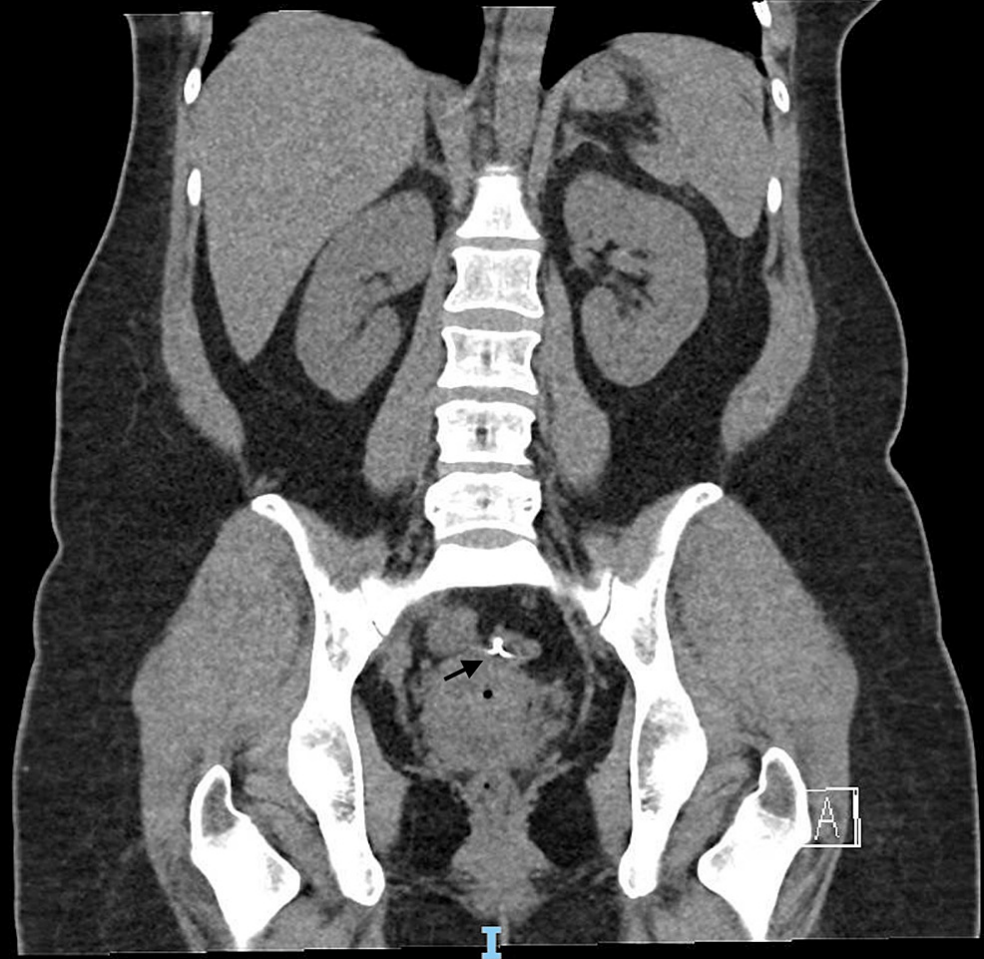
A CT scan of the KUB (coronal view) shows the intrauterine device (arrowed) has migrated and is now located between the body of the uterus and the adjacent rectosigmoid colon. KUB: kidney, ureter, and bladder
